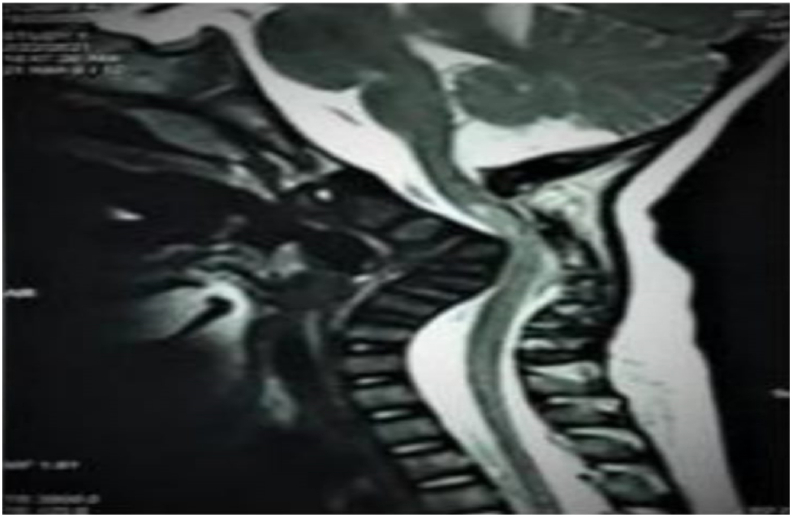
Cervical spine MRI, Sagittal T2 sequence, showing kyphotic deformity of the cervical spine causing significant cord compression and T2-hyperintense signal abnormality related to myelomalacic changes.
